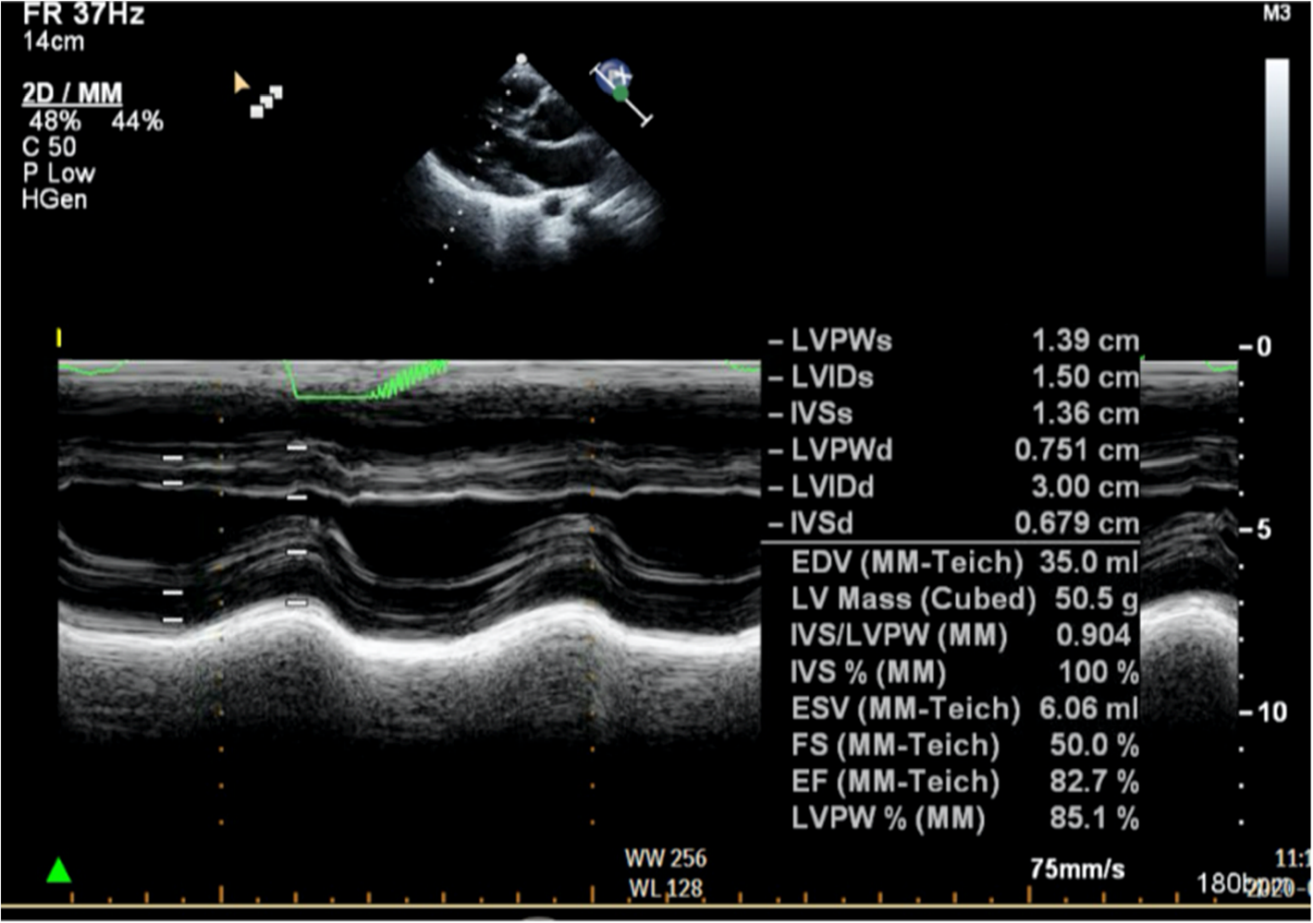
Echocardiography study showed normal systolic and diastolic function with no pericardial effusion
